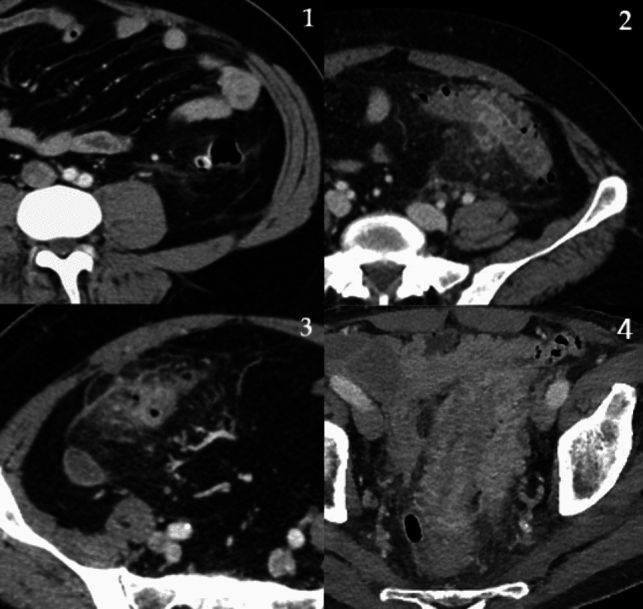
Axial CT showing peridiverticular inflammation grades: (1) Minimal: rare fine threads of high attenuation. (2) Mild: multiple threads of high attenuation that remain distinct, vessels are clearly visible. (3) Moderate: many threads difficult to resolve individually, vessels difficult to discern. (4) Severe: dominant pattern of increased attenuation in the fat could be mistaken for fluid collection, vessels not visible
